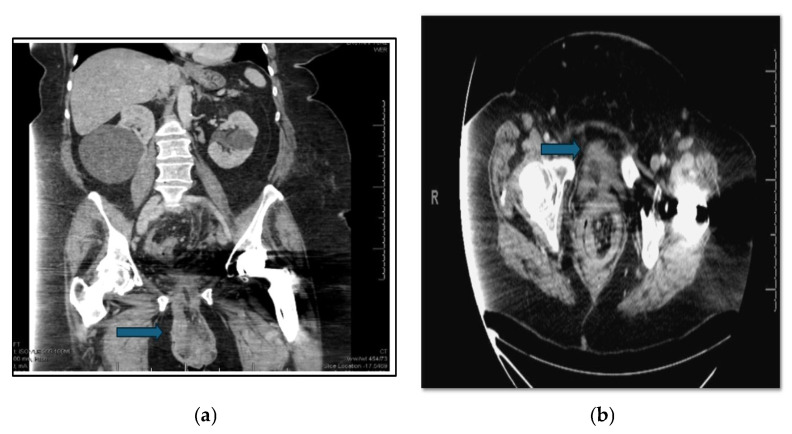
(a) Axial CT scan of the abdomen and pelvis demonstrates prolapsed loops of large bowel in the vagina. The blue arrow indicates eviscerated bowel. (b) Coronal view CT abdomen and pelvis scan, and blue arrow showing the prolapsing loops of large bowel.
